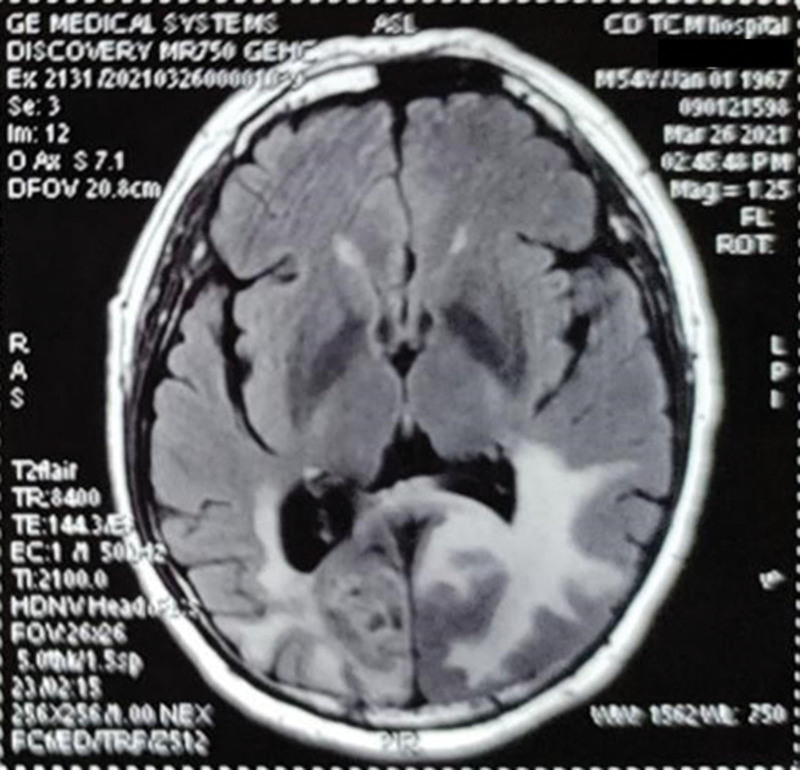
In March 2021, MRI indicated a smaller size at 3.2 × 3.4 × 4.5 cm of the lesion. MRI = magnetic resonance imaging.
